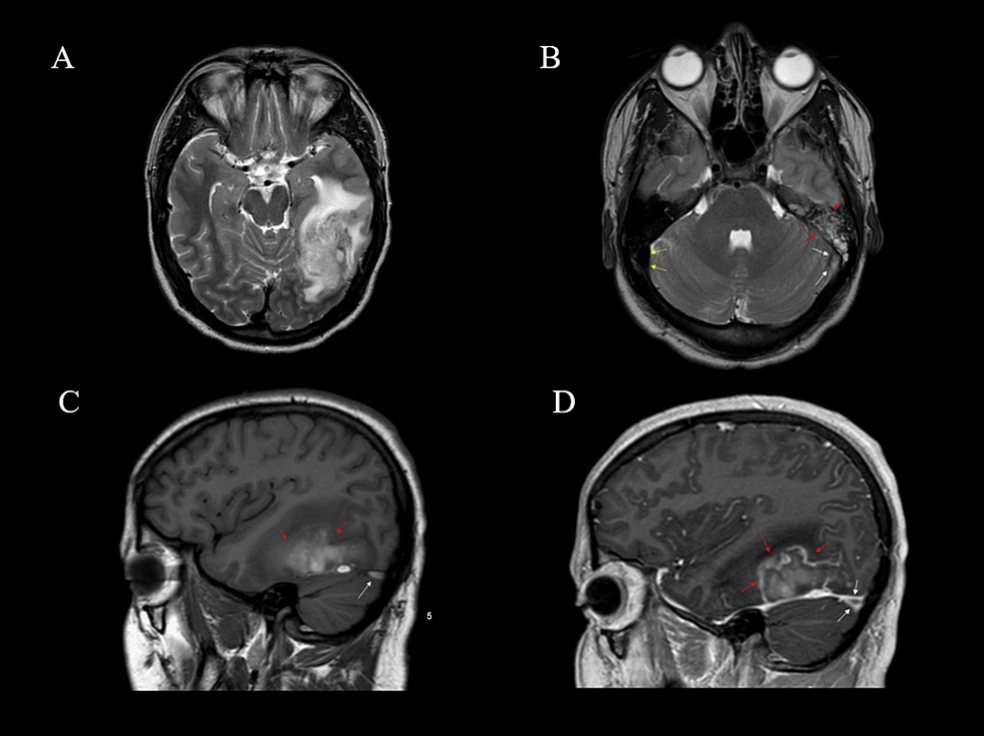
Brain magnetic resonance imaging (A) Axial T2WI: shows the extent of the venous infarction within the left occipital lobe. (B) Axial T2WI: white arrows show loss of flow voids at the distal left transverse sinus related to the thrombus, and red arrows show an associated left mastoid air cells effusion. On the other hand, yellow arrows show the normal flow void at the distal right transverse sinus. (C) Sagittal T1WI pre-gadolinium: white arrow shows T1 hyper-intense thrombus within the left transverse sinus, and red arrows show within the left occipital lobe infarction in keeping with the hemorrhagic nature of the infarction. (D) Sagittal T1WI post IV gadolinium injection: white arrows show an intraluminal filling defect within the left transverse sinus consistent with acute venous sinus thrombosis, and red arrows show a left occipital lobe hemorrhagic infarction with minimal irregular peripheral enhancement.
